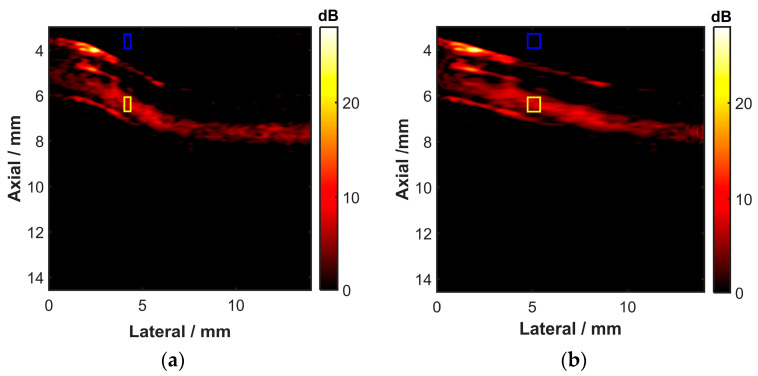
Flow image information calculated with power Doppler: (a) original image without motion compensation and (b) the compensated image. The blood flow area (yellow rectangular area) and the clutter area (blue rectangular area) were used to calculate the CNR.
